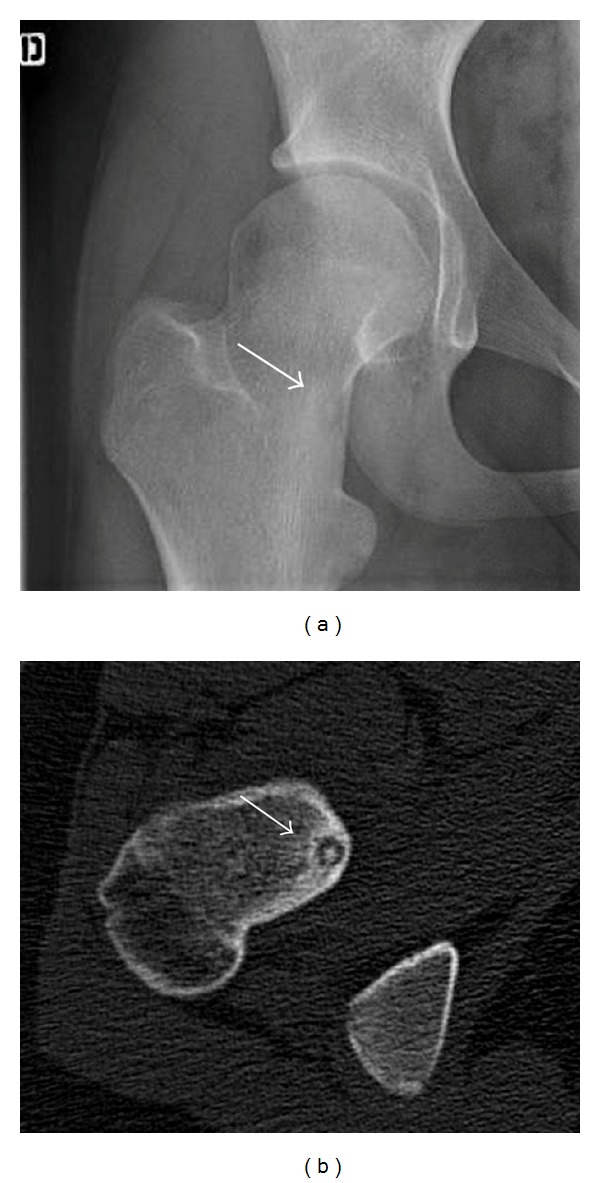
Same patient as in Figure 1. (a) A repeated frontal X-ray of the right hip, performed six months later, demonstrates a radiolucent nidus of femoral neck. (b) Confirmation was obtained by CT-scan.
